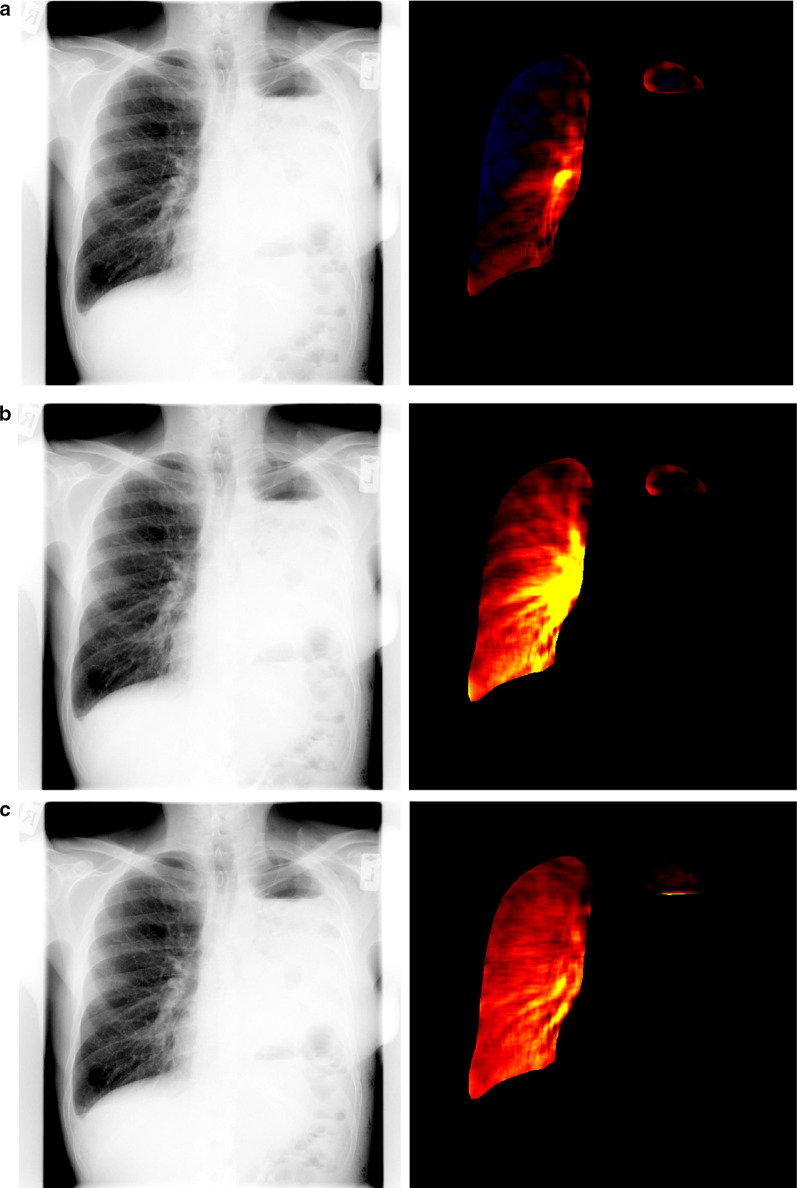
Demonstration of use of dynamic chest radiography to detect pulmonary blood flow. DCR in a male in his 50 s following left pneumonectomy. Standard DCR images is shown in lef-thand panels, DCR perfusion mapping in right. Still images prior to (a), during (b) and after (c) right ventricular contraction
